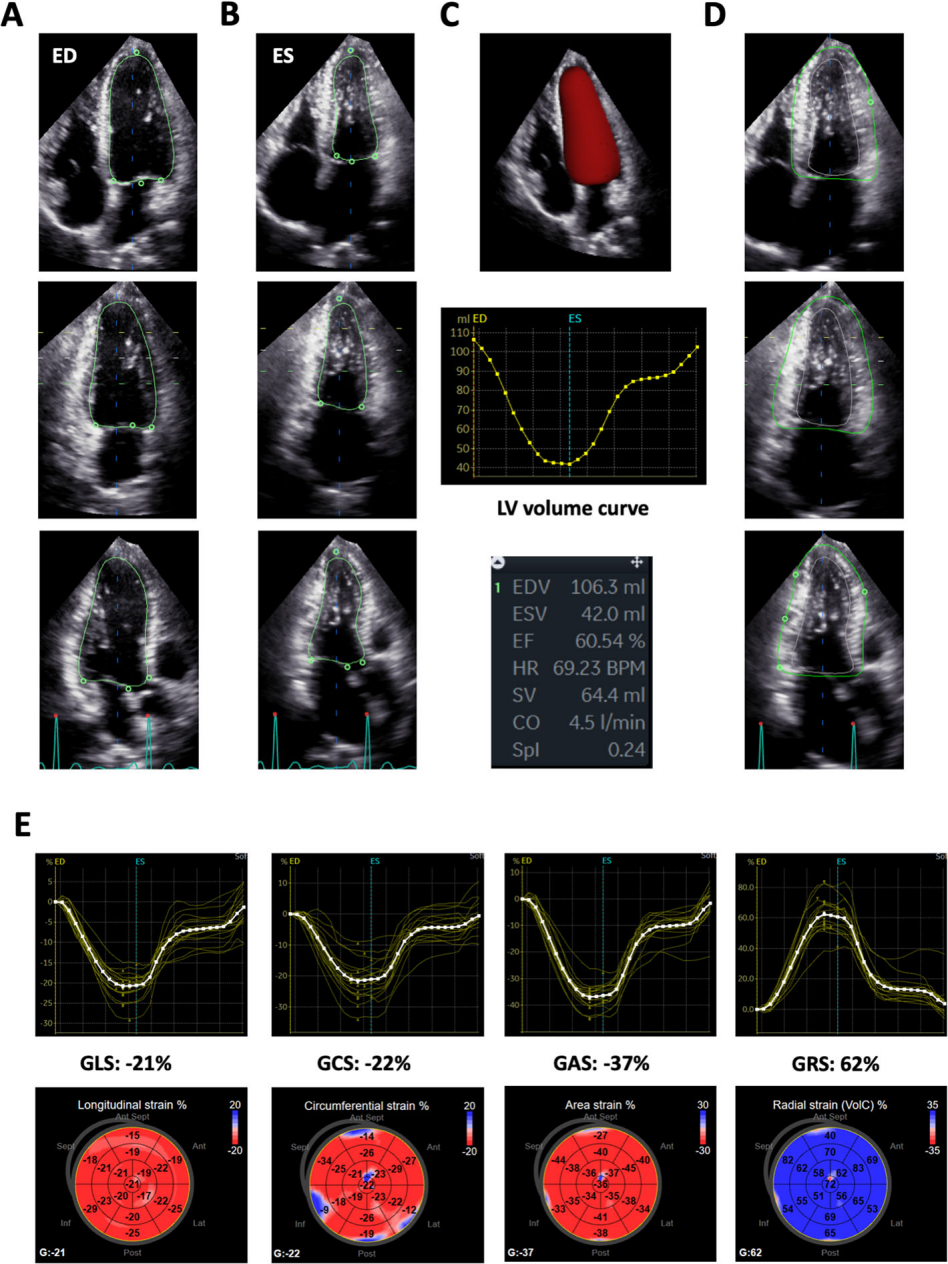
3D strain analysis using GE software in a healthy subject. Left ventricular endocardial border determination in apical 4-, 2-, and long-axis views at end-diastole (a) and end-systole (b). Green lines indicate the endocardial border. The software performed LV border determination during one cardiac cycle, and the generated LV volume curve, from which LV end-diastolic volume, end-systolic volume, and LV ejection fraction (LVEF) were calculated. A beutel of the left ventricle is also shown (c). Epicardial border determination with manual editing identified the region of interest for subsequent speckle tracking (d). 3D regional and global longitudinal strain curves, a circumferential strain curve, an area strain curve, a radial strain curve, and a bull’s eye map of corresponding regional strains (e), generated with 3D speckle tracking analysis. CO, cardiac output; ED, end-diastole; EDV, end-diastolic volume; EF, ejection fraction; ES, end-systole; ESV, end-systolic volume; HR, heart rate; GAS, global area strain; GCS, global circumferential strain; GLS, global longitudinal strain; GRS, global radial strain; Spl, sphericity index; SV, stroke volume
